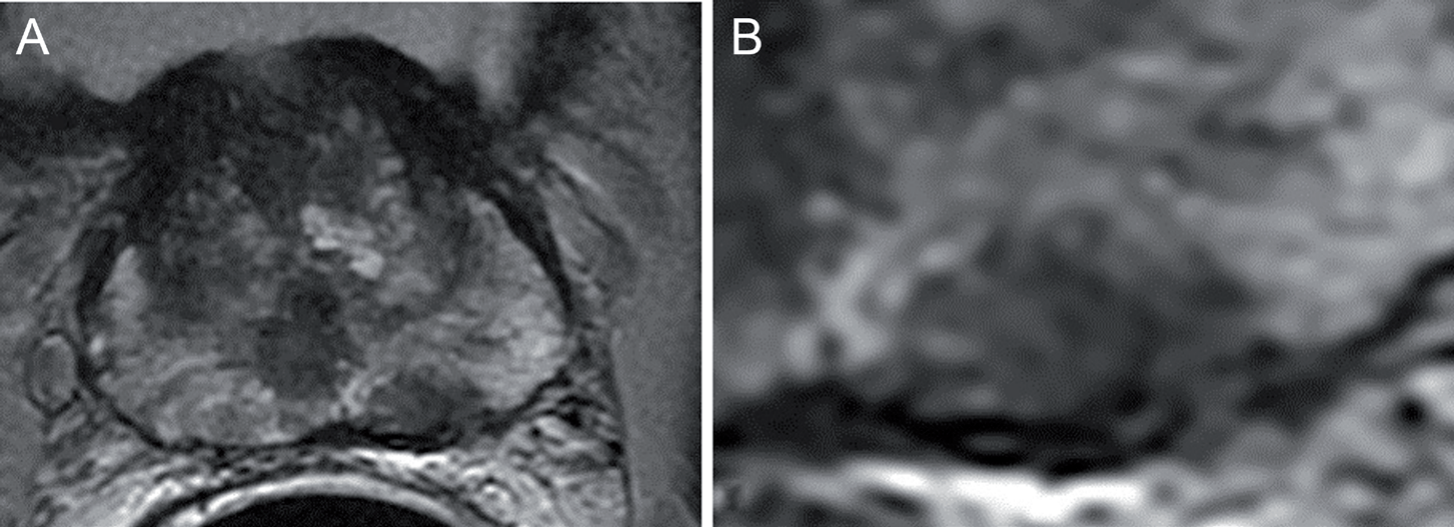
Magnetic resonance images of axial sequences T2WI (A, B) of interrupted capsule. A tumor alteration hypointense exists in the left mid-basal posterior paramedian peripheral zone. In B, we can see better how the capsular profile, represented by a thin hypointense line on the left in the area of contact with the lesion, is interrupted.
